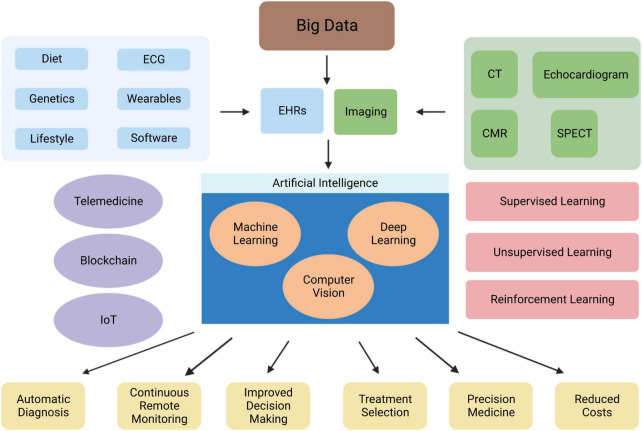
Overview of the role of AI in cardiovascular medicine. Abbreviations: EHRs, electronic health records; CMR, cardiac magnetic resonance; CT, computed tomography; IoT, internet of things; SPECT, single photon-emission computerised tomography.
